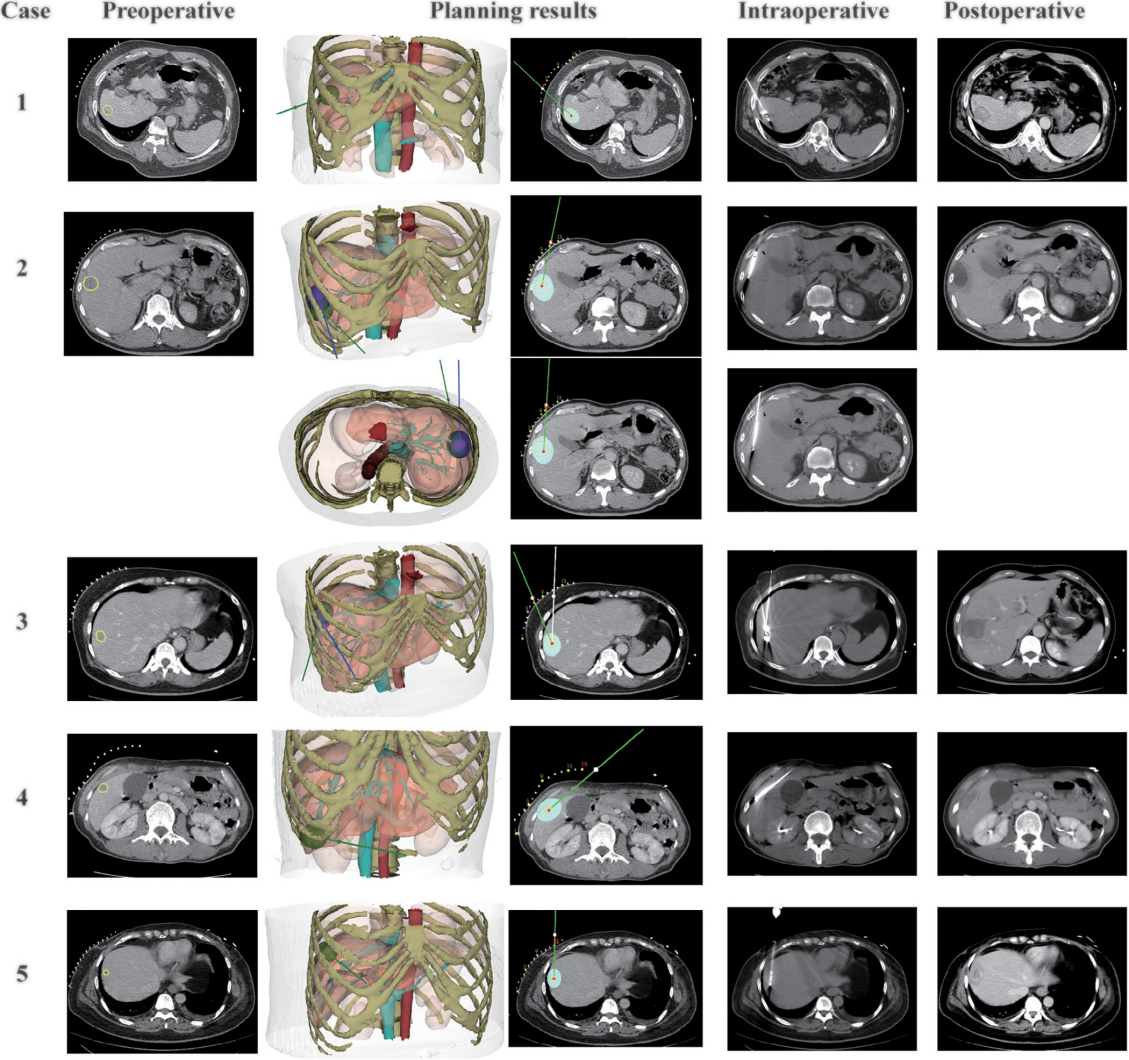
The planning results of clinical cases. Preoperative CT images demonstrate the target tumor segmented by the yellow line. The planning results include 3D visualization and 2D projection on the axial CT images of the generated plans. The intraoperative and postoperative images show the actual needle trajectory and ablation regions.
